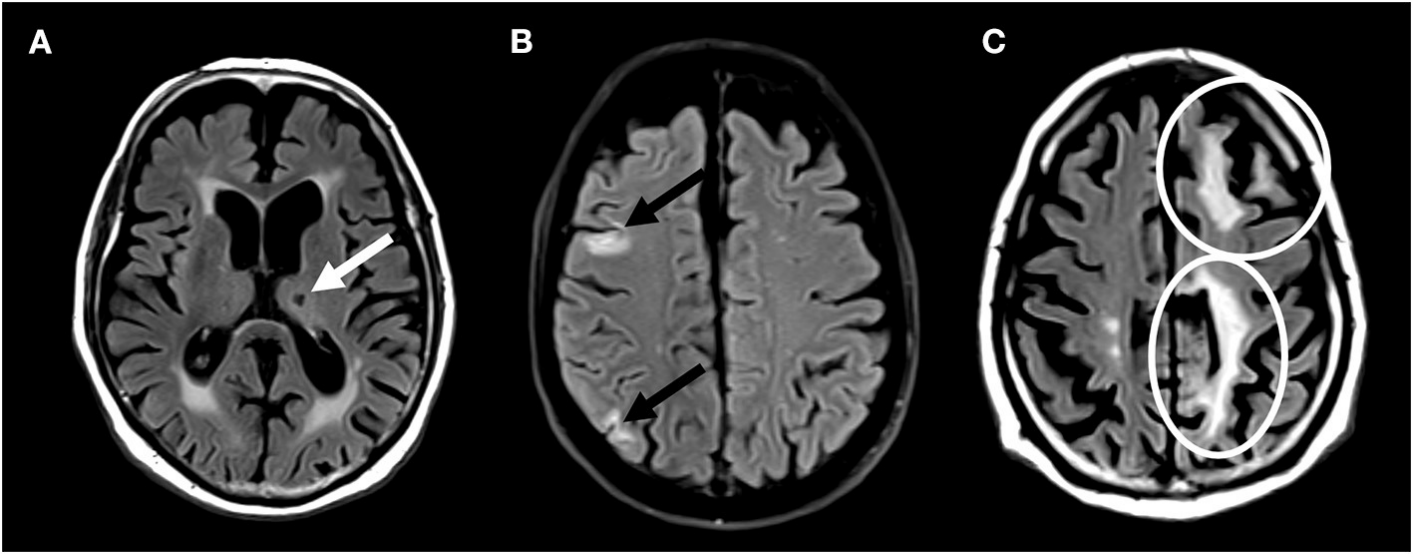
Brain magnetic resonance imaging showing (A) small non-cortical infarct (B) small cortical infarcts, and (C) large non-cortical and cortical infarcts.
